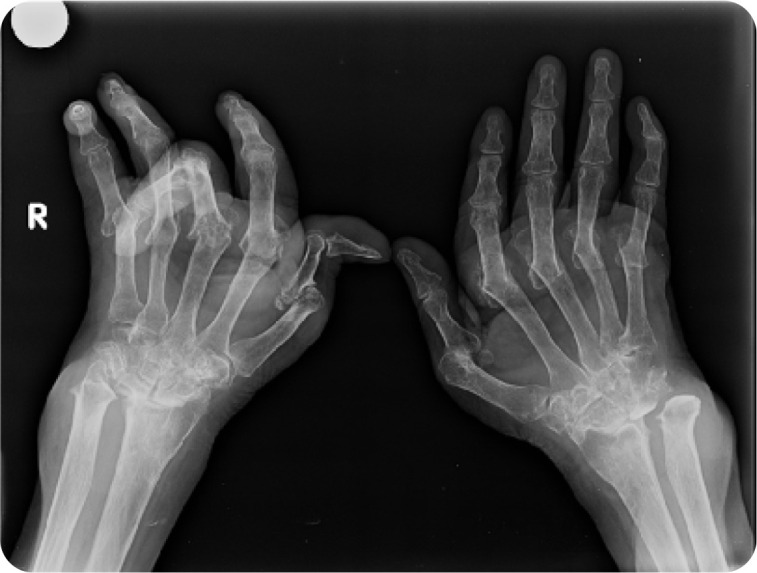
(above). Hands and wrists x-ray of the same patient. Note the severe destructive changes affecting all carpal bones, all metacarpophalangeal and proximal interphalangeal joints bilaterally. Severe subluxations of metacarpophalangeal, proximal interphalangeal and distal interphalangeal joints, along with cysts formations are evident.
